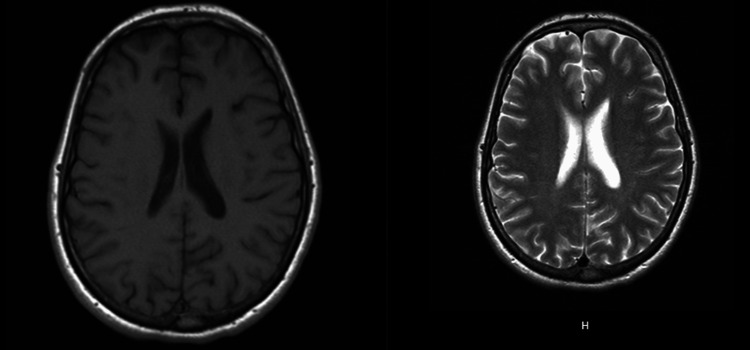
Axial T1-weighted image showing a subtle hypointense focus in the left parietal white matter Corresponding T2-weighted image demonstrating hyperintense signal in the same region. Together, these findings are suggestive of an area of focal demyelination
